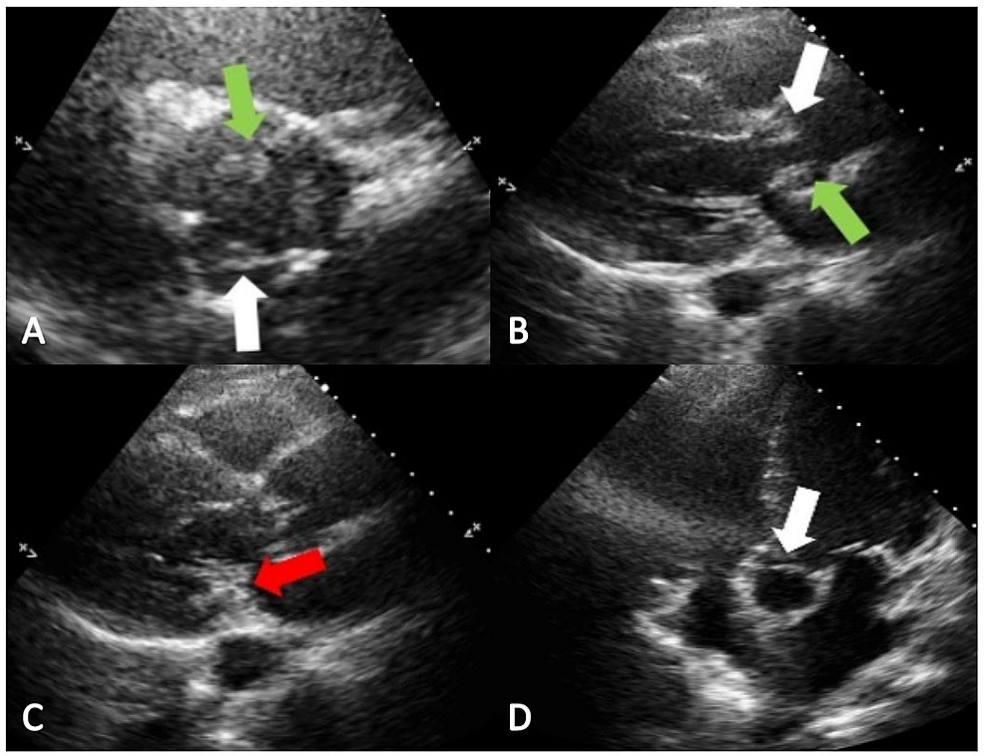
Transthoracic echocardiogram: (A) Parasternal-short axis view shows mobile hypodensity in the noncoronary cusp (green arrow) and in the right coronary cusp (white arrow); (B) Parasternal-long axis view shows mobile hypodensity in the noncoronary cusp (green arrow) and in the right coronary cusp (white arrow); (C) Parasternal-long axis view that shows mobile hypodensity in the posterior mitral leaflet (red arrow); (D) Apical five chamber view shows mobile hypodensity in the right coronary cusp (white arrow)
